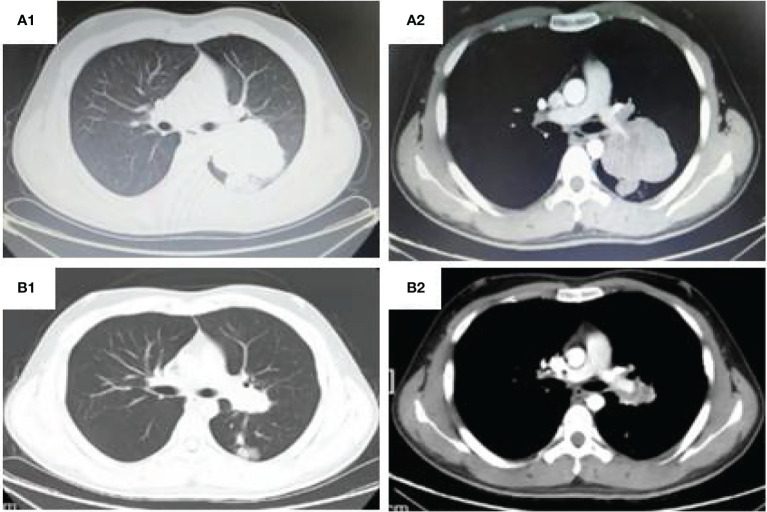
CT thorax: (A1, 2) (Shanxi Provincial Cancer Hospital,2021-02-03) shows a large irregular and well-defined border mass lesion measuring 7.7×6.3 cm with inhomogenous enhancement beside the left hilus pulmonis across the interlobar fissure and an enlarged mediastinal lymph node measuring 2.7×1.9 cm. (B1, 2). (Shanxi Provincial Cancer Hospital,2021-05-12)Tumor was remarkable reduced to 2.5*3.4cm and the mediastinal lymph node was 1.2 cm.
